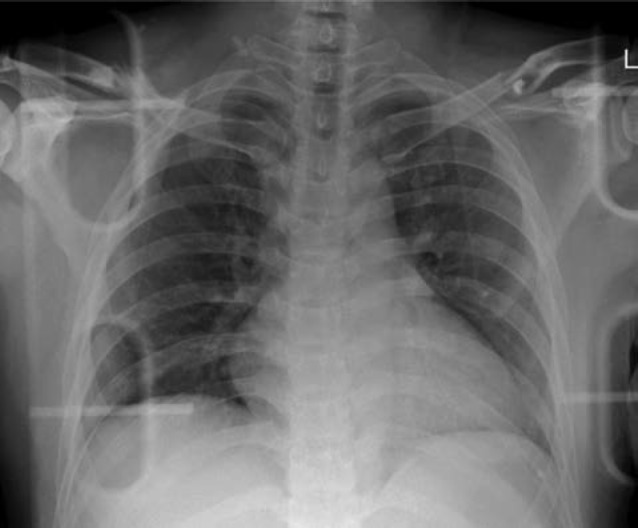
Bilateral clavicles fracture with no other injury seen. The radiograph was taken during patient was stabilized with arm board.
